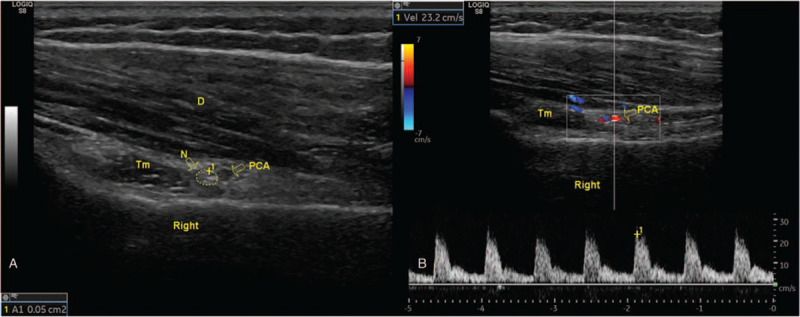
Three months after the treatment, the musculoskeletal ultrasound showed that the axillary nerve swelling disappeared, the cross-sectional area was 0.05 cm^2^ (A), and the blood flow velocity of the PCA returned to 23.2 cm/s (B). PCA = posterior humeral circumflex artery, Tm = Teres minor.
